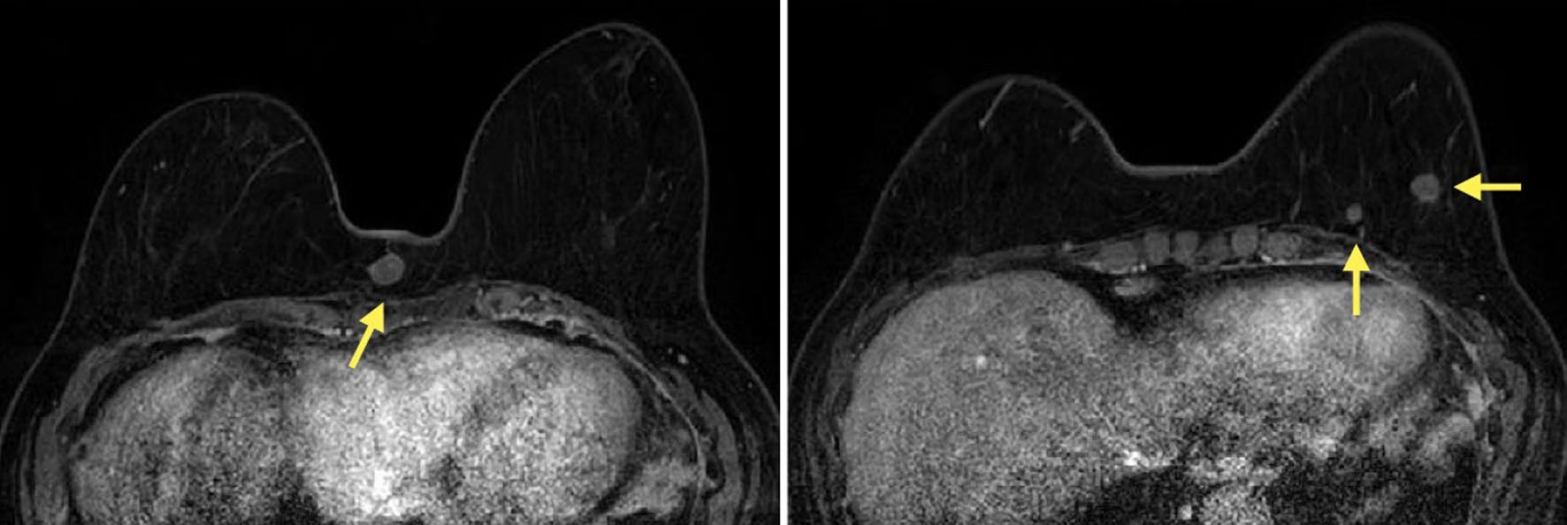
Contrast-enhanced MRI showed multiple masses with rim enhancement and hypo-diffusion in both breasts’ subcutaneous and mammary tissues
